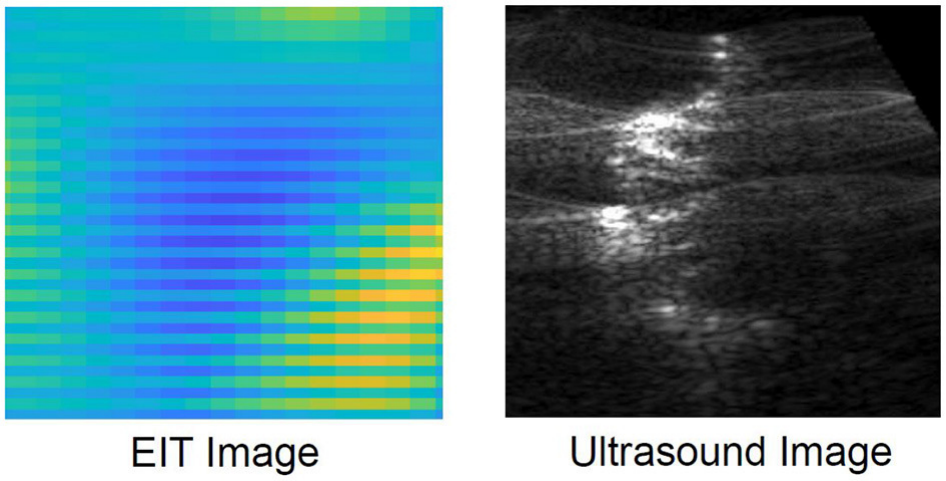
The EIT image for scenario 5 is compared against its ultrasound image.
